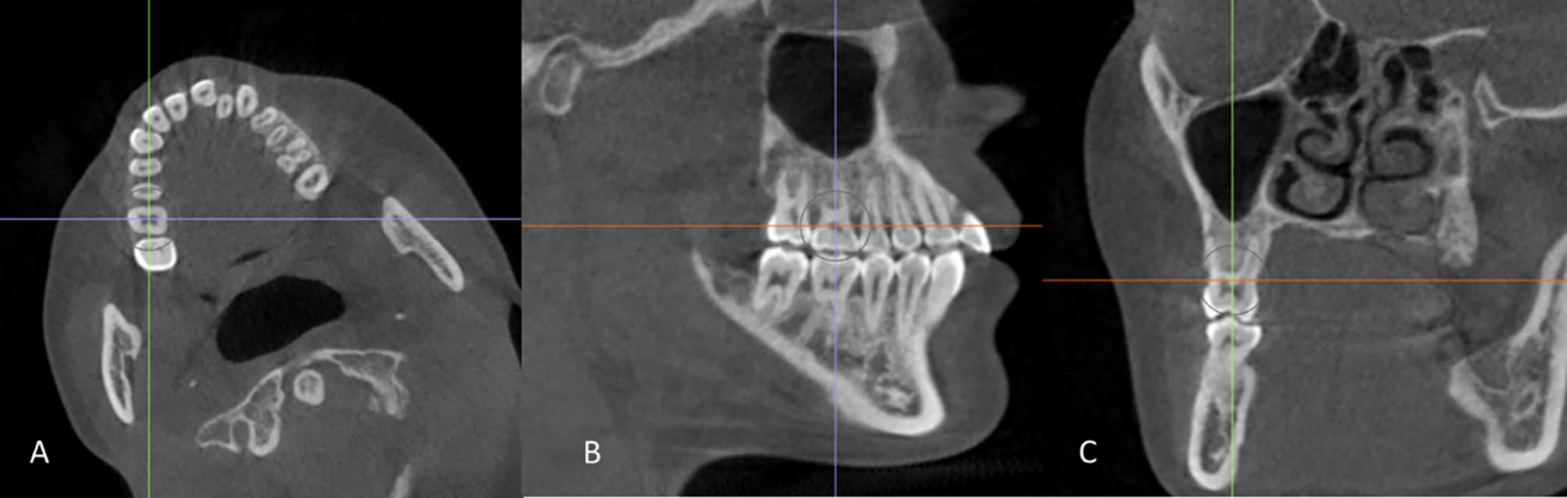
Steps of CBCT orientation with intersection of the coronal (blue), and axial (red) planes; A Orientation of the sagittal view of the dental arch was done so that the long axis of the intended tooth is perpendicular to both coronal and sagittal coordinate. B and C, Adjustment of the axial coordinate so that it crosses the other coordinate at the center of the intended tooth
